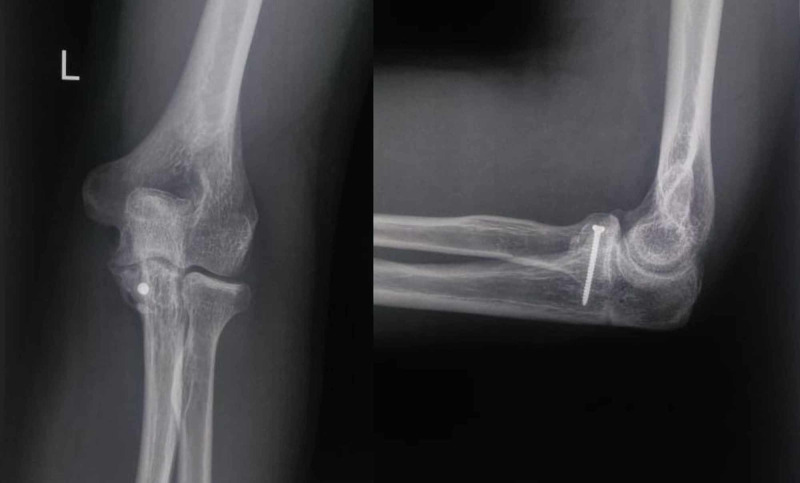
Case 5 - anteroposterior radiograph and lateral radiograph of the elbow at the final follow-up showing reduced elbow with the united coronoid fracture
